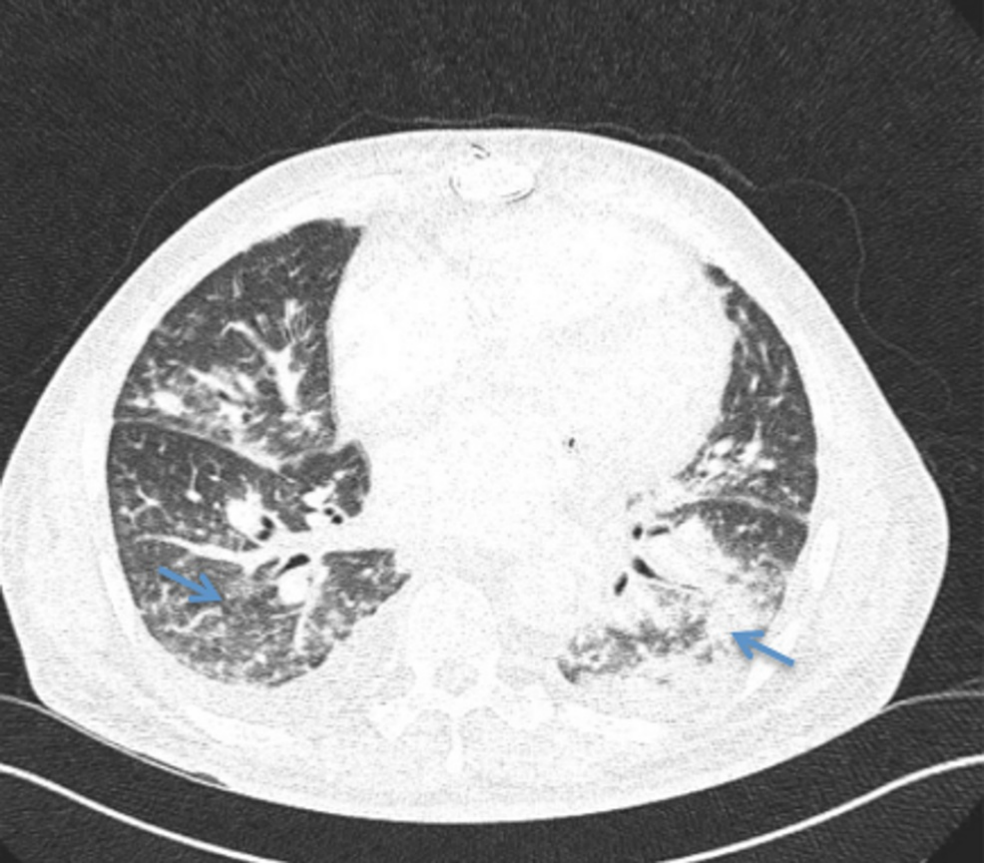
Computed tomography scan images of the chest. Diffuse nodular infiltrates (blue arrows).
